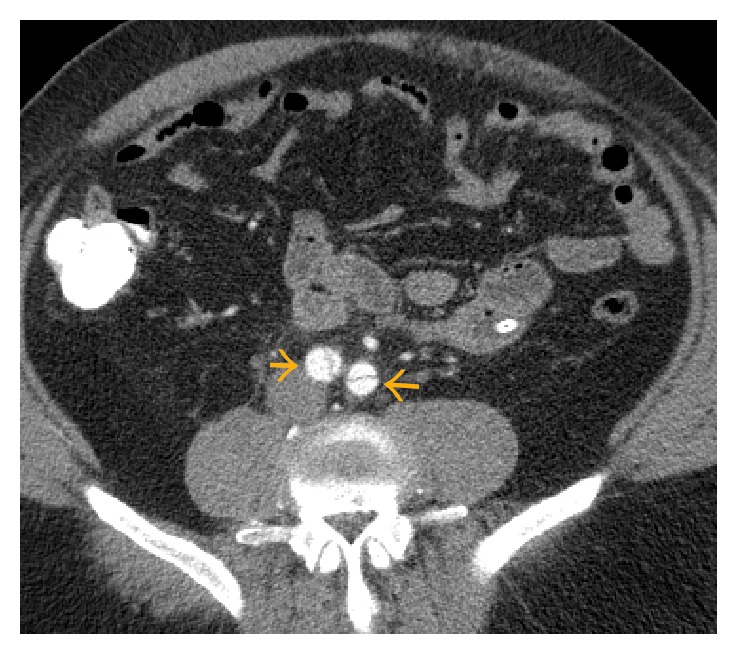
CT angiography showing acute type B aortic dissection extending into the proximal common iliac arteries (yellow arrows). This parallels with the patient's clinical complaints of scrotal pain and dysuria.
